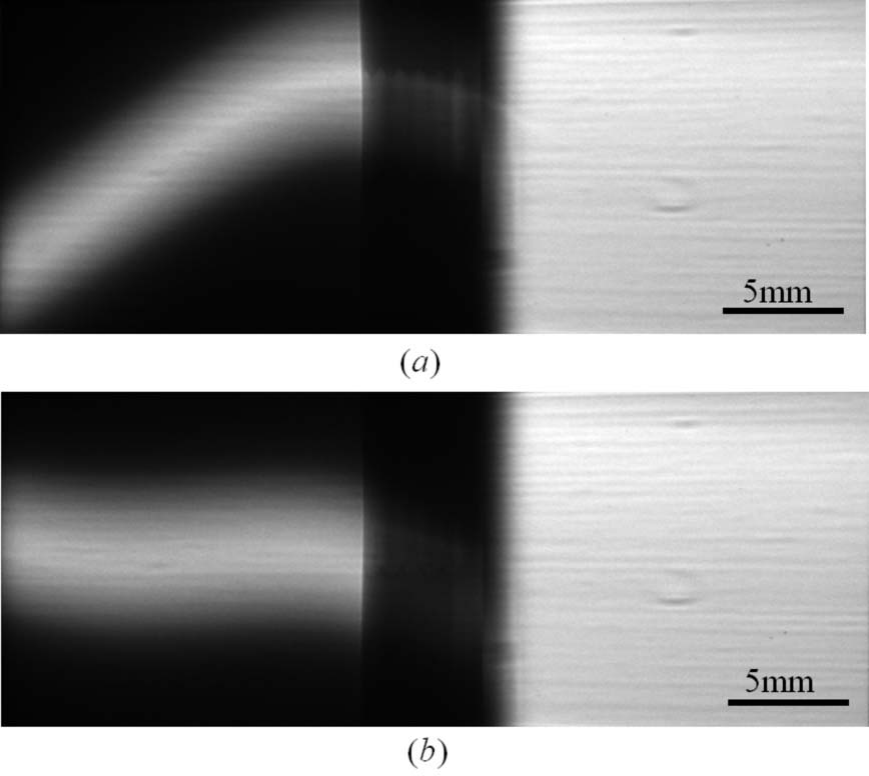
(*a*) Two X-ray beams without a sample. Left: reflected beam. Right: direct beam. (*b*) Two X-ray beams after correction for the oblique shape of the reflected beam.
